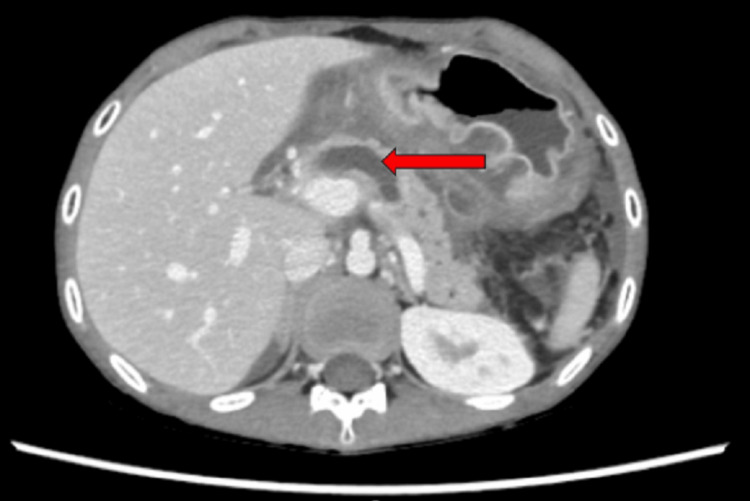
CT scan showing dilated pancreatic duct (arrow) CT: computed tomography
